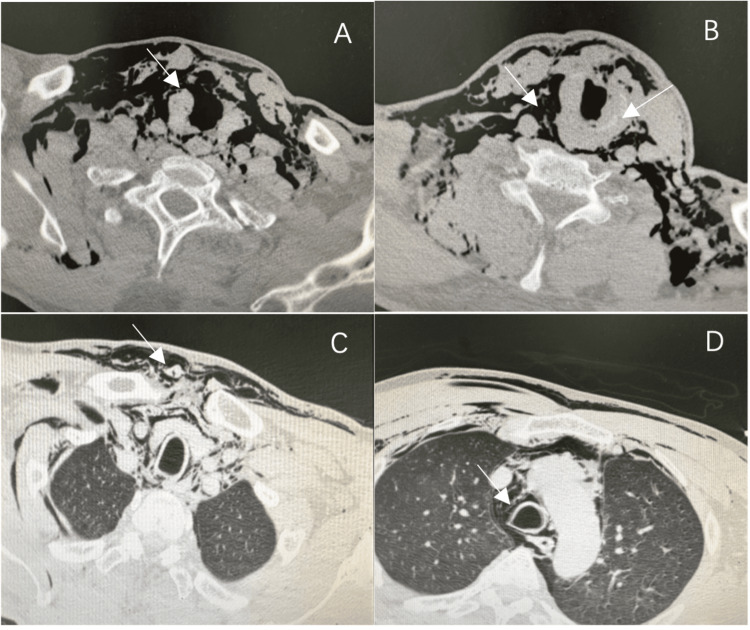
Preoperative CT imaging of the neck and chest (A) Airway CT demonstrating an irregular contour and deformity of the subglottic tracheal lumen. (B) CT showed a linear lucency through the cricoid cartilage, suggestive of cartilage fracture, with extensive subcutaneous emphysema in the cervical soft tissues. (C) Chest CT showing subcutaneous emphysema along the anterior chest wall. (D) Chest CT demonstrating pneumomediastinum with free air tracking along the mediastinum
